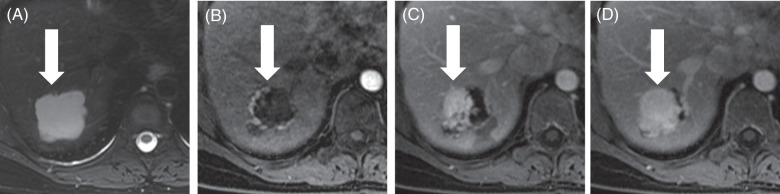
Hepatic hemangioma—Characteristic features on MRI with contrast. A 53-year-old woman with a hepatic hemangioma (arrow) undergoes MRI with extracellular contrast. (A) Axial T2-weighted images show a lobulated, circumscribed, and moderately T2-hyperintense lesion. (B) After injection of contrast, axial T1-weighted images in the arterial phase show that the lesion has peripheral, nodular, and discontinuous areas of enhancement. The signal intensity of these enhancing areas is similar to that of the aorta, that is, blood pool. (C) In the portal venous and (D) delayed phases, the areas of enhancement increase centripetally and continue to match the blood pool.
